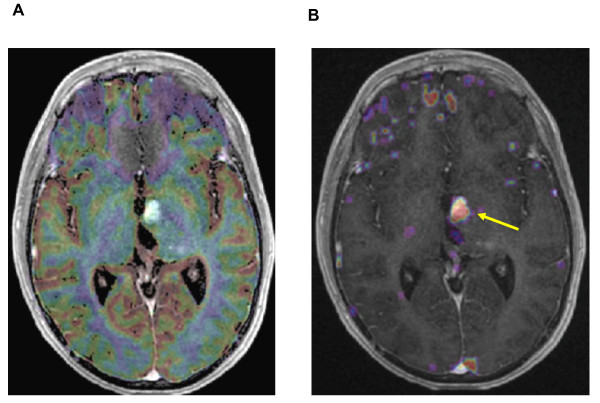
Six months after irradiation: superimposed MR images with T1-weighted sequence after iv Gadolinium injection and co-registered Perfusion (left) and Permability maps (right). [A] Gradient-echo axial image with color overlay map showing no focus of hyperperfusion in regard of the strong contrast enhancement [B] The superimposed Permeability map with a strong microvascular leakage (yellow arrow) strictly corresponding with the enhanced area in the T1-weighted sequence.
